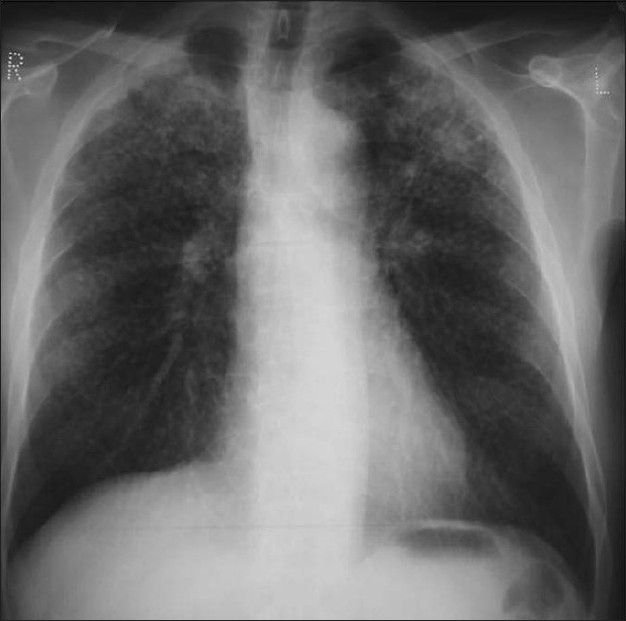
A chest radiograph shows reticulonodular shadowing with bilateral apical lung fibrosis and high density nodules in coal workers pneumoconiosis
